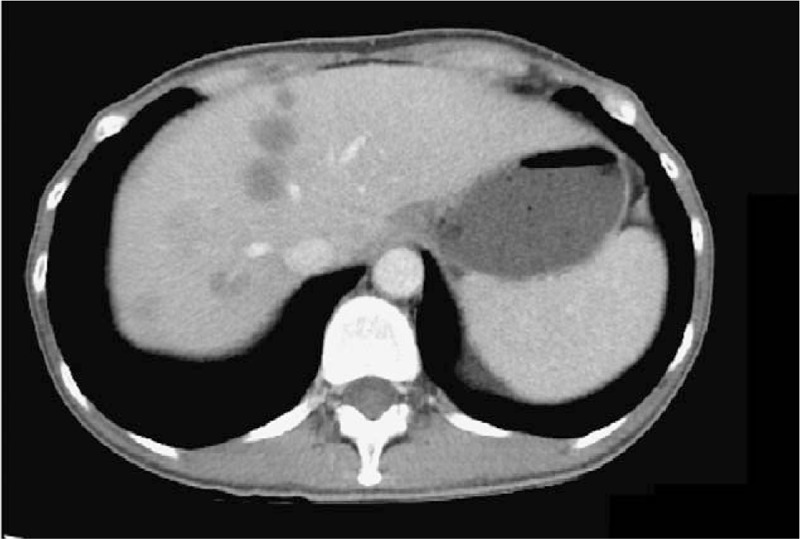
CT scan upon diagnosis of breast metastasis revealed disease progression (progressive disease by RECIST 1.1 criteria^[47]^). There were increased hepatic lesions, and the largest hepatic lesion was 1.6 cm.
